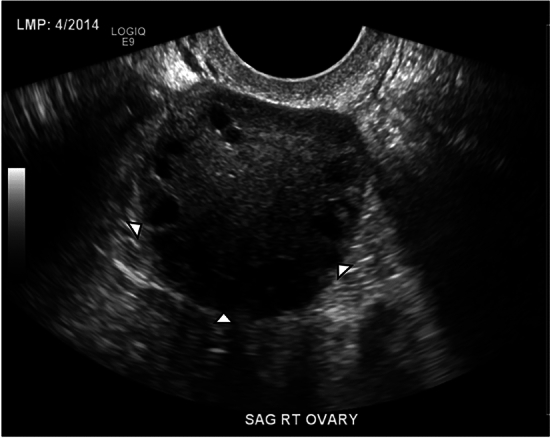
A 28-year-old woman with no significant past medical history presented with irregular menstrual periods. She underwent pelvic ultrasound which demonstrated mildly enlarged ovaries with numerous peripherally oriented small follicles (arrowheads). The right ovary measured 22 mL in volume, while the left ovary measured 16 mL (not shown)
